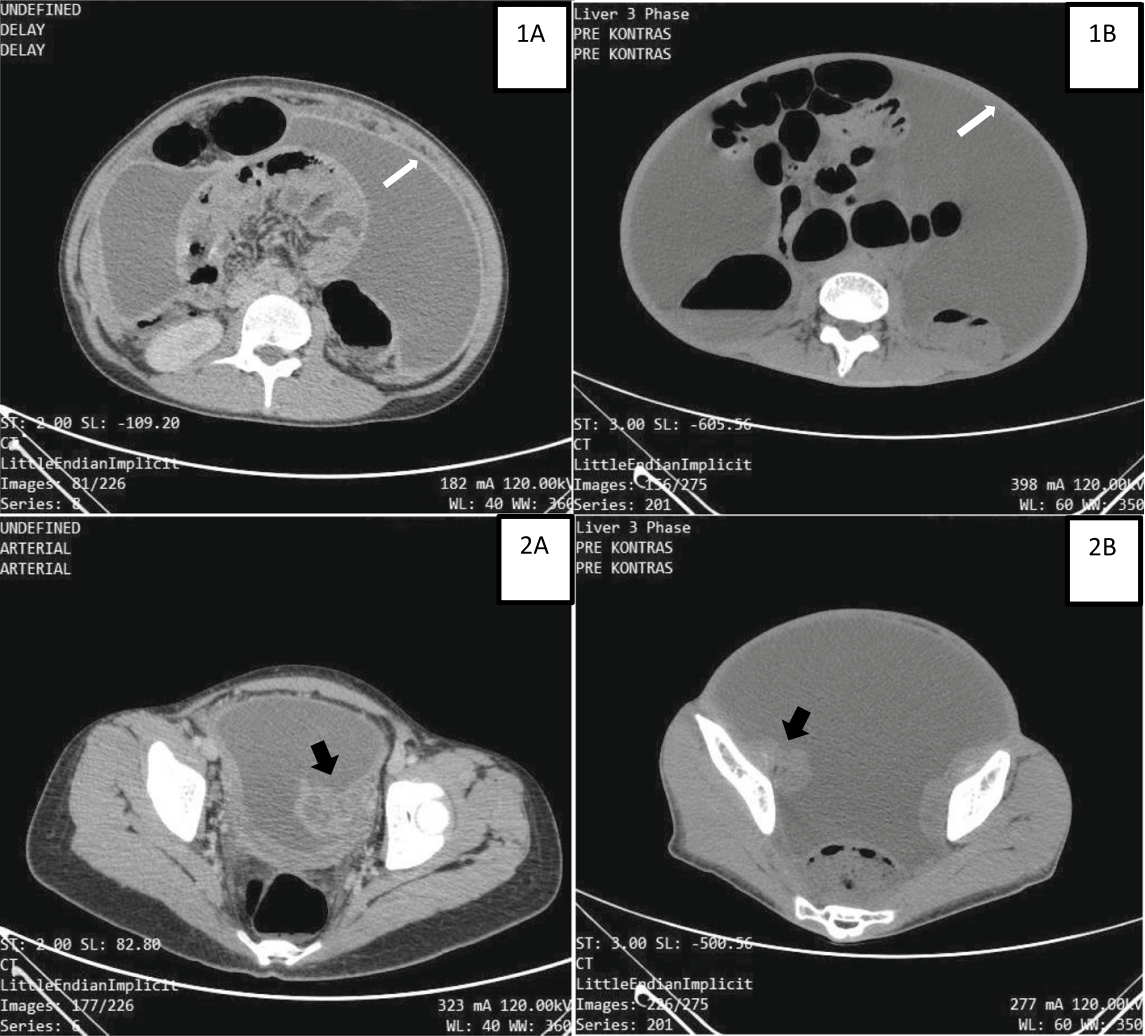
Abdominal CT scan in Patient 1 and Patient 2. Both showed significant amount of ascites (white stars) with smooth non-nodular parietal peritoneum thickening (white arrows). Hypo-isodense multiloculated cystic mass of adnexa previously interpreted as ovarian tumor (black arrows) in Patient 1 and 2
